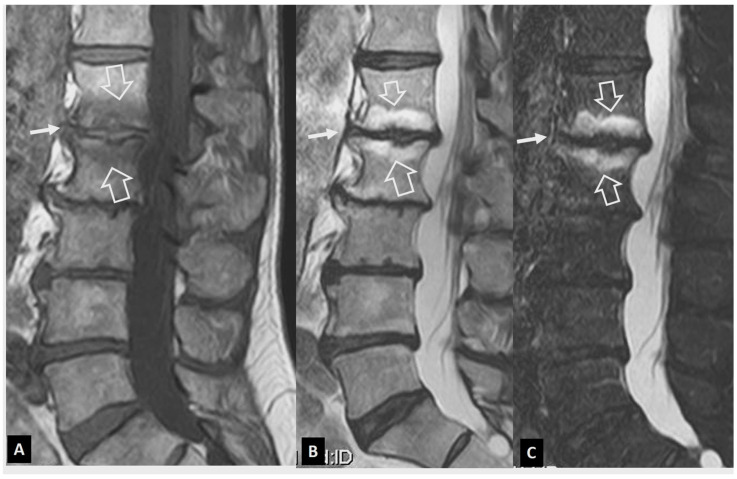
A 66-year-old male patient with a long history of scoliosis and non-specific low back pain. Sagittal T1-w (A), T2-w (B), and STIR (C) MR images show a dehydrated L1–L2 disc, with severe height reduction (thin arrows) and associated bone marrow edema (open arrows) in keeping with MODIC type I changes.
